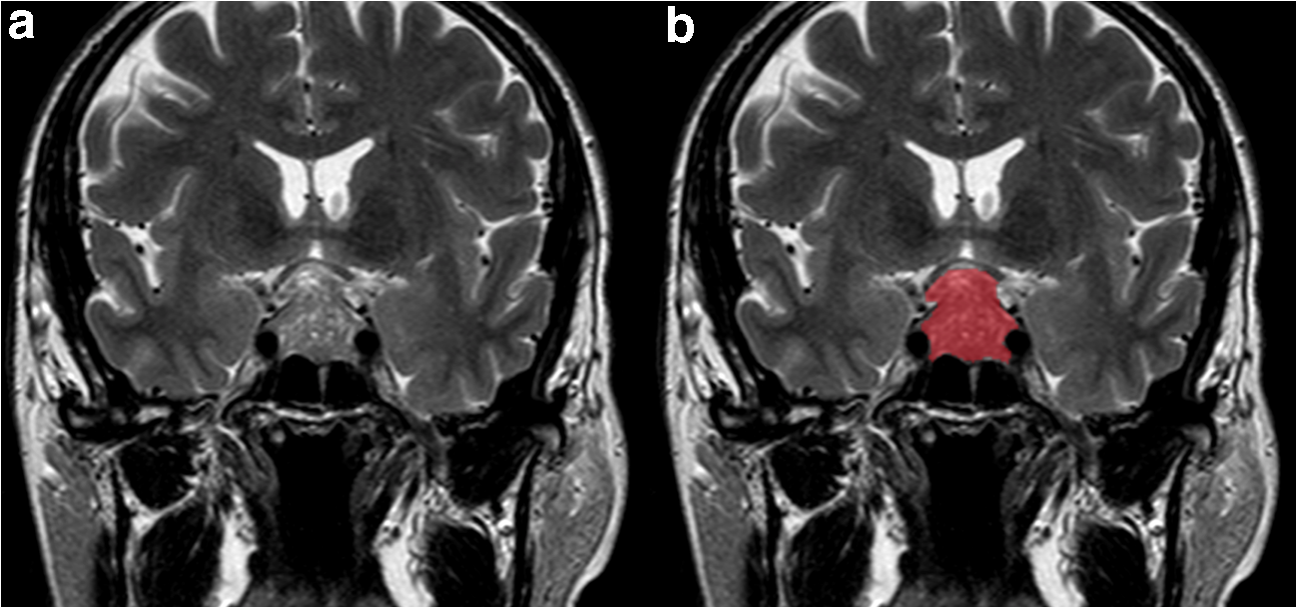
Pituitary macroadenoma segmentation example on coronal T2-weighted (a), showing hand-drawn ROI placement (b)
